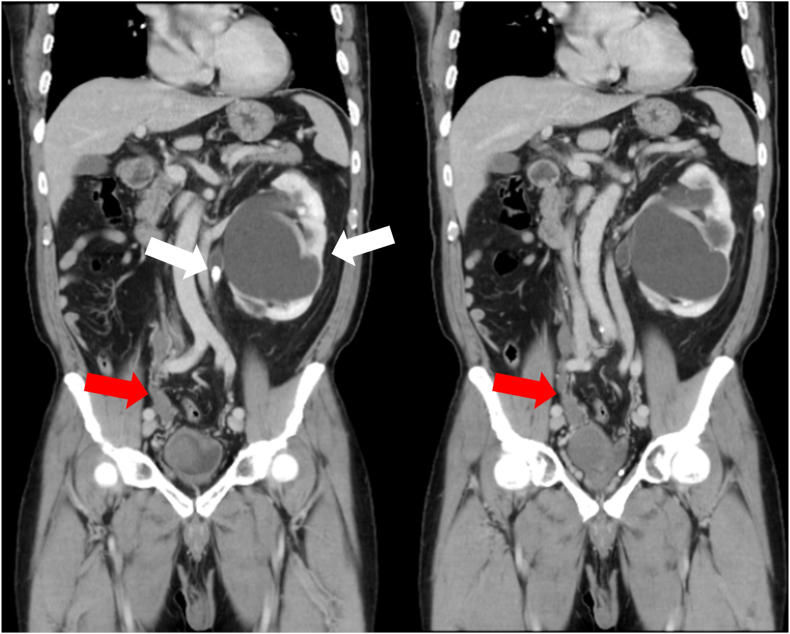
Coronal CT images revealed a left UPJ stone with moderate left hydronephrosis (white arrow) and nonvisualization of the normal right kidney. Two consecutive coronal images also revealed a dilated residual right ureter connecting to a right seminal vesicle cyst (red arrow).
